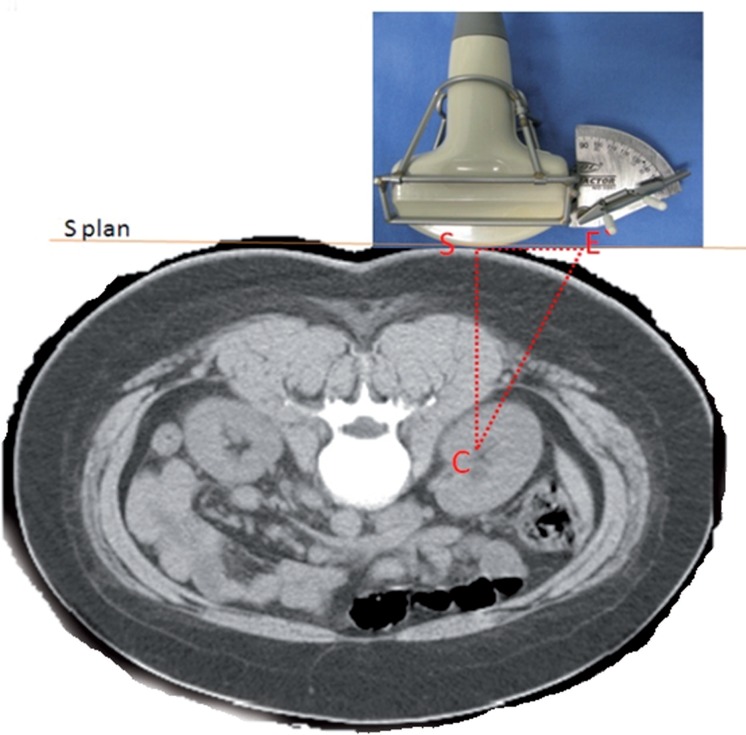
vertices of the initial right triangle: the target calyx (c); the “apex” of ultrasound transducer in contact with the skin, perpendicular to the aimed calyx (s); and the orthogonal projection of the center of the protractor in the skin plane (E').
